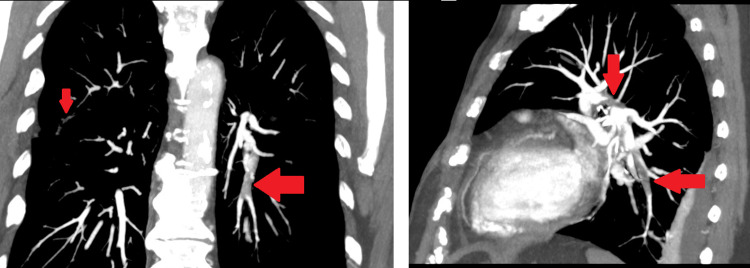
Coronal and sagittal chest CT showing bilateral pulmonary embolism (arrows)
